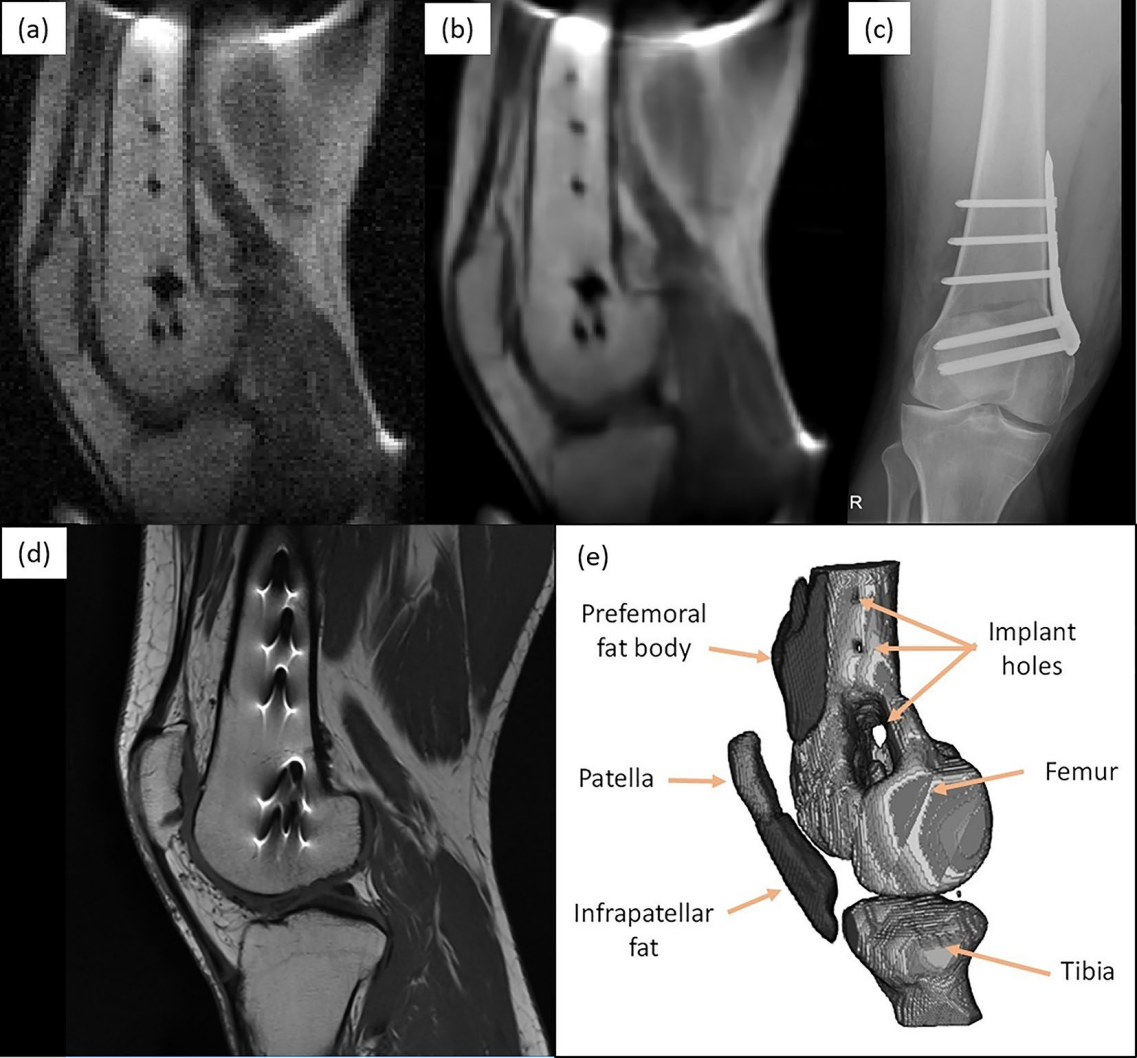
Images of fixation metallic implant attached to the femur, consisting of a plate and seven screws: (a) sagittal view of a raw low-field image acquired with the 72 mT system (9 mm slice from $$T_1$$-weighted 3D-RARE acquisition with in-plane resolution of $$1.3\times 2$$ mm$$^2$$, 12 min scan time, eight years after femoral shaft osteotomy); (b) same, but BM4D-filtered^27^ and rescaled by $$\times 2$$ to increase the number of pixels; (c) lateral X-ray computed radiography (two weeks after surgery); (d) sagittal view of the same knee, acquired with a Siemens Skyra 3 T system ($$T_1$$-weighted 2D-RARE acquisition with slice thickness 3.9 mm and pixel resolution $$0.26\times 0.26$$ mm$$^2$$, one year after surgery); and (e) 3D reconstruction from $$T_1$$-weighted 3D-RARE acquisition with isotropic resolution of 2 mm, 20 min scan time, where selected muscle and fat segments have been removed (eight years after surgery).
